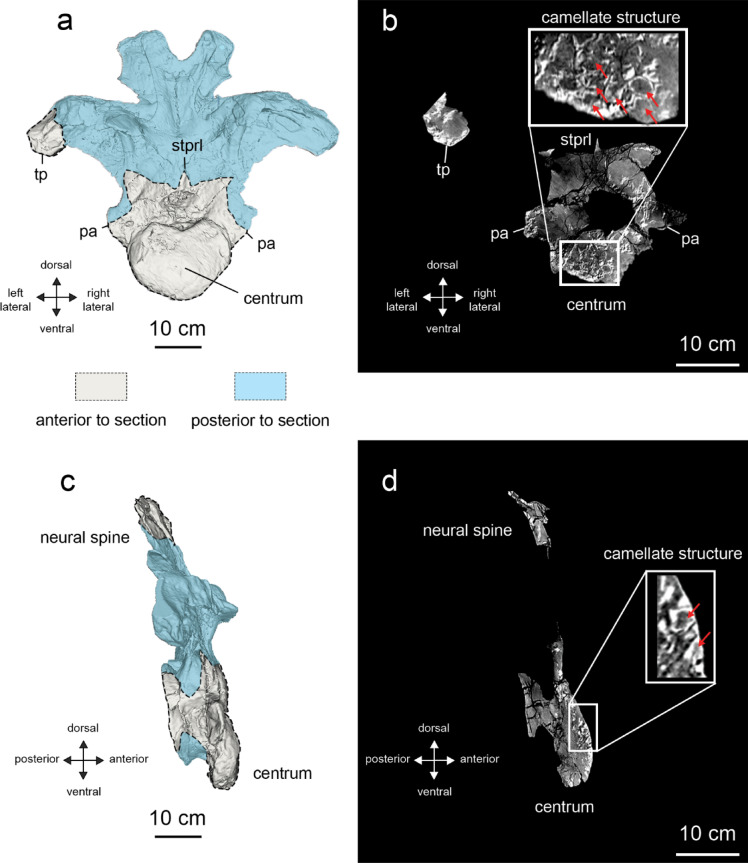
CT scan of the anterior dorsal vertebra of *Uragasaurus kalasinensis* (holotype PRC 460). Three-dimensional reconstructions of the vertebra in anterior view (a) and right lateral view (c). Corresponding CT sections in anterior view (b) and right lateral view (d). The section plane corresponds to the dashed line separating the grey (anterior) and blue (posterior) regions in the 3D reconstructions. White boxes highlight camellate pneumatic cavities within the centrum. Red arrows indicate polygonal camellae within the camellate internal structure of the centrum. Black-and-white arrows indicate anatomical orientation in each panel.
